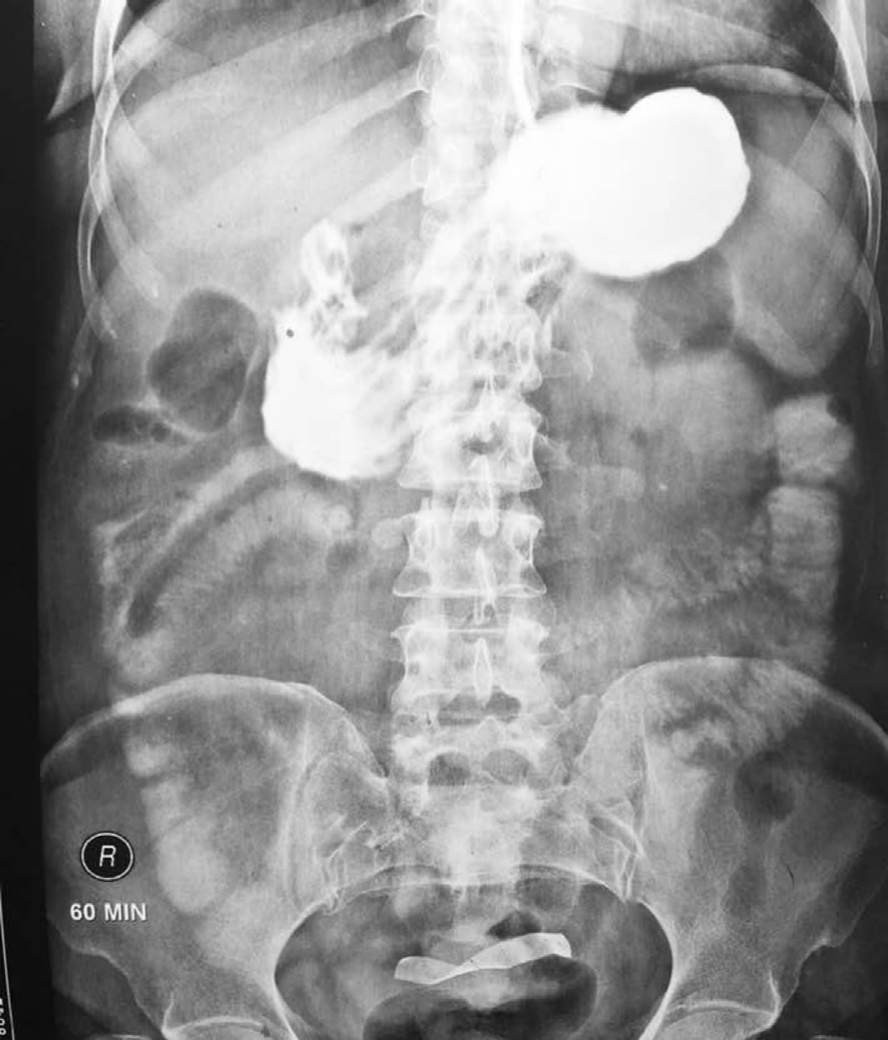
X-ray at 1 hour showing gauze in terminal small gut.
